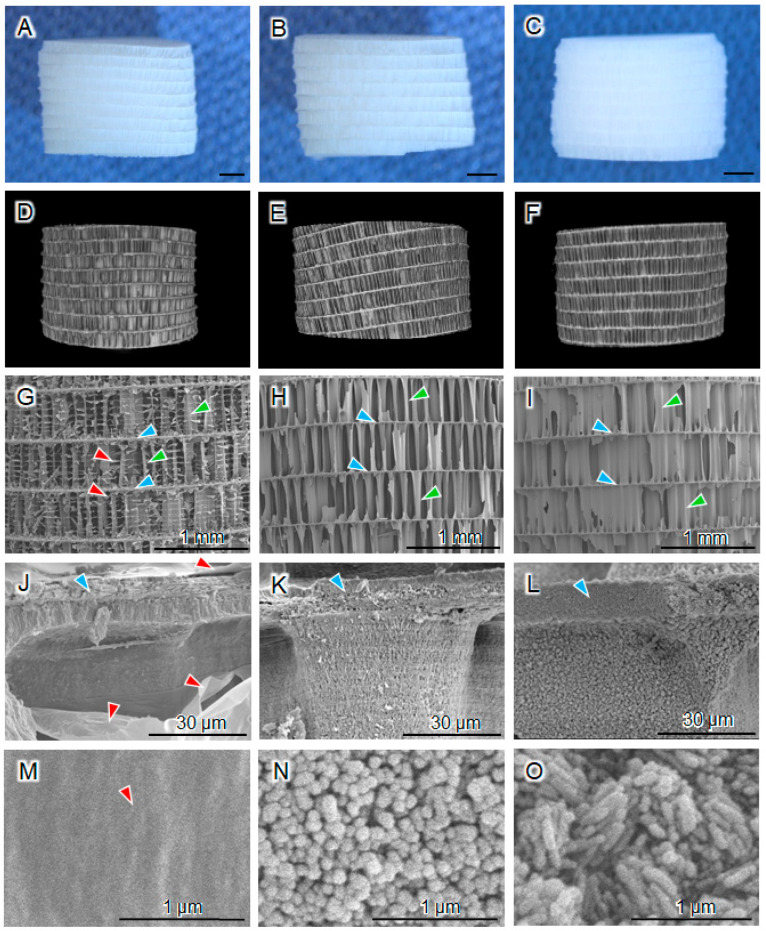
(A–C) Gross images, (D–F) µ-CT images, (G–L) SEM images, and (M–O) high-magnification SEM images of raw CB, NaClO-treated CB, and CAp-CB scaffolds. Scale bars: (A–I) 1 mm, (J–L) 30 µm, and (M–O) 1 µm. The green, blue, and red arrowheads indicate the walls, lamellar septa, and organic layer, respectively.
